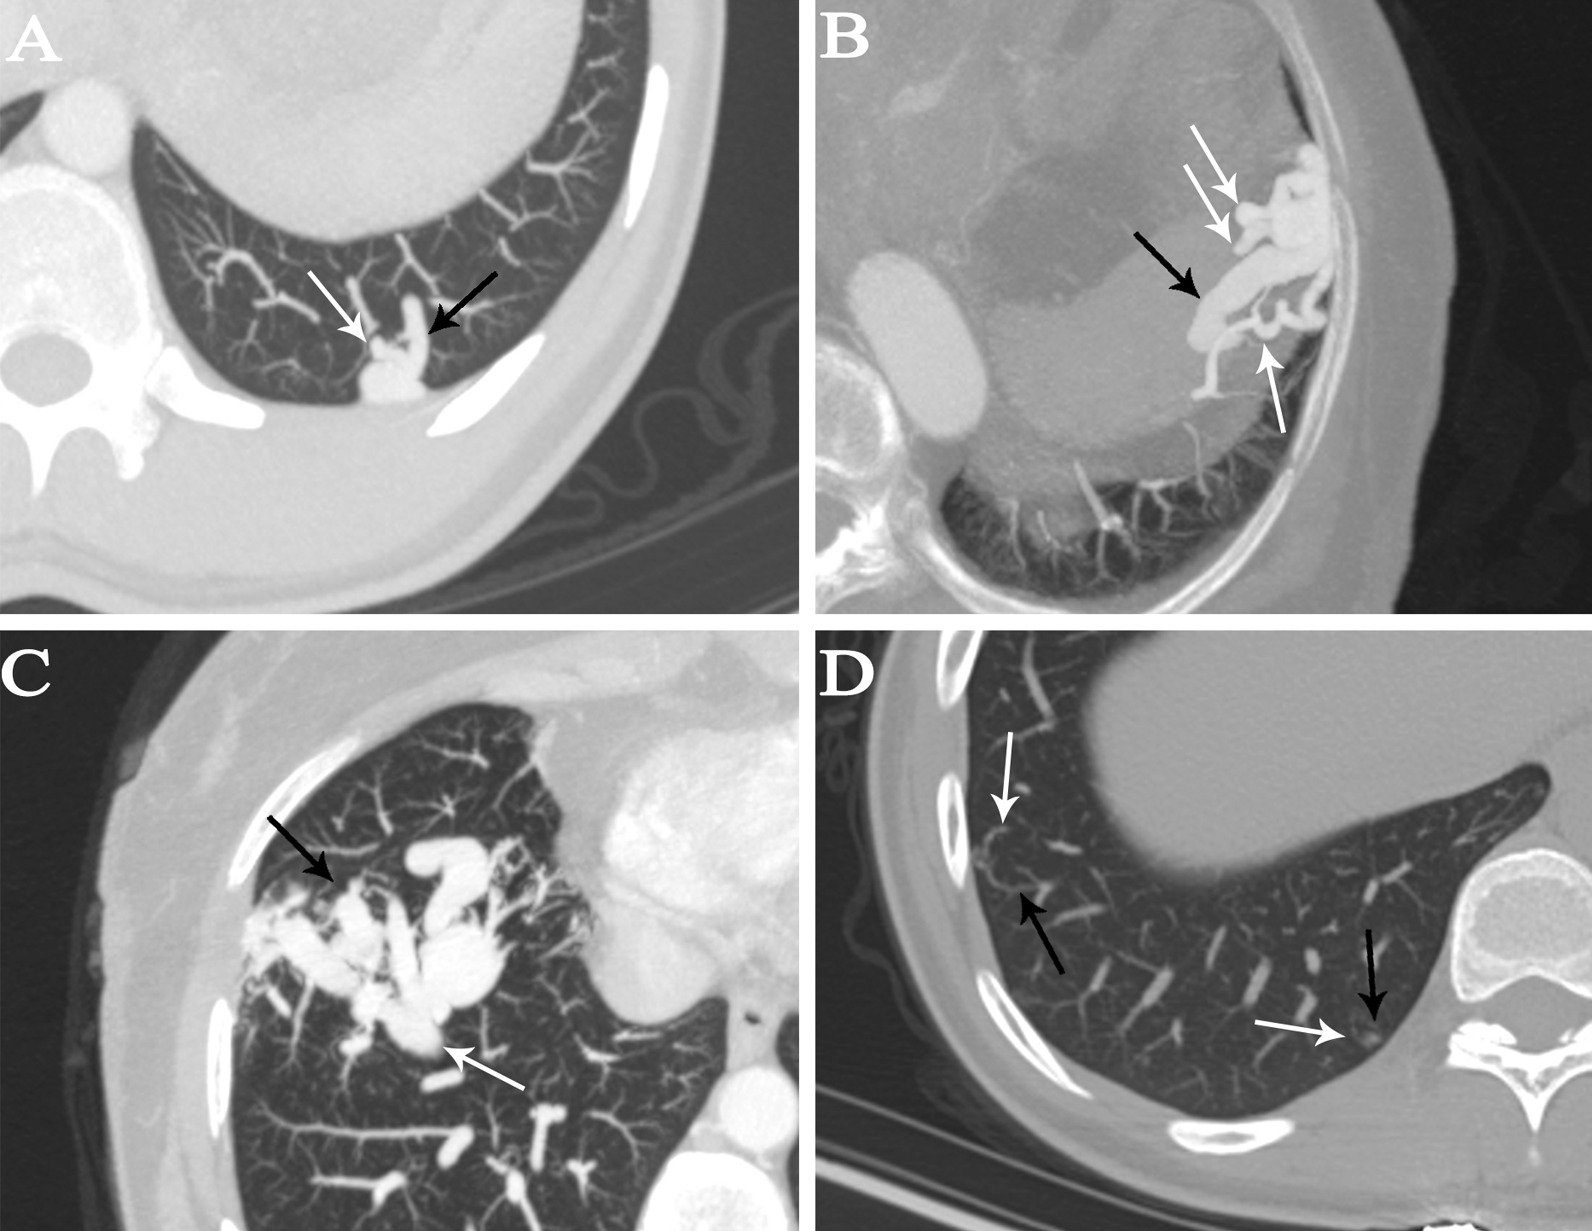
All types of PAVMs were detected by MSCT and CTA. A The simple type of PAVM consisted of one feeding artery (white arrow) and one draining vein (black arrow). B Three feeding arteries (white arrows) and one enlarged draining vein (black arrow) were detected in one complex PAVM. C One diffuse PAVM had multiple feeding arteries involving a large part of the parenchyma (black arrow) and one enlarged draining vein (white arrow). D Telangiectatic PAVMs with one feeding artery (white arrows) and one draining vein (black arrows) were detected by MSCT. *PAVMs* pulmonary arteriovenous malformations, *MSCT* multi-slice spiral computed tomography, *CTA* computed tomography angiography
